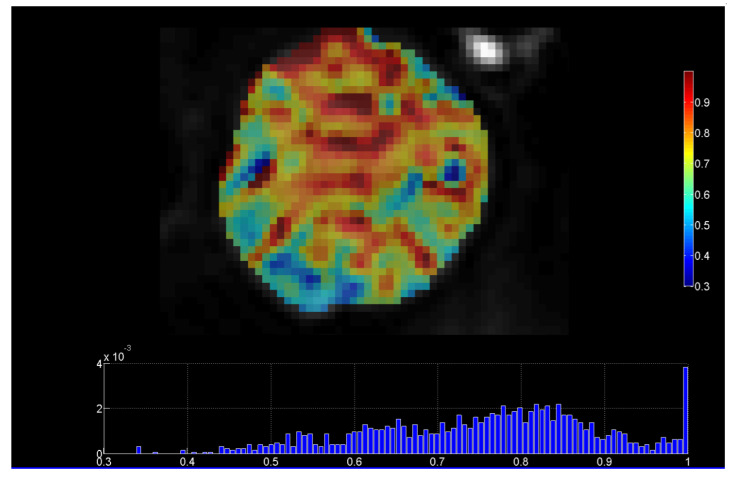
DWI-MRI stretched-exponential (SEM) DWI-MRI parametric map, revealing highly heterogeneous parts of a dedifferentiated liposarcoma (with permission from the department of Medical Imaging, Heraklion University Hospital). Heterogeneity index α ranges from 0 to 1, with lower values of α indicating microstructural heterogeneity.
